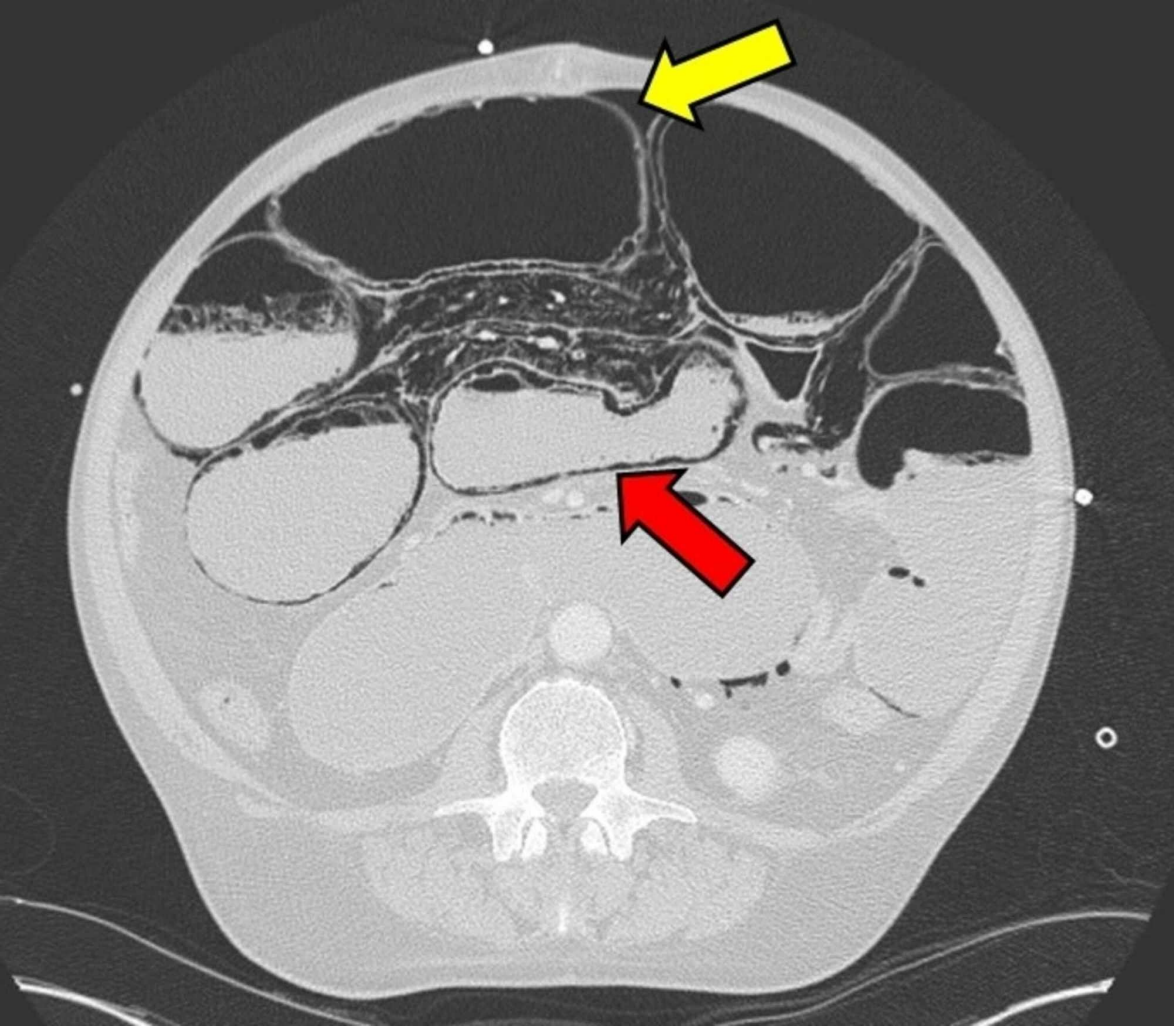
CT scan of the abdomen showing pneumatosis intestinalis (red arrow) and free intraperitoneal air (yellow arrow).
